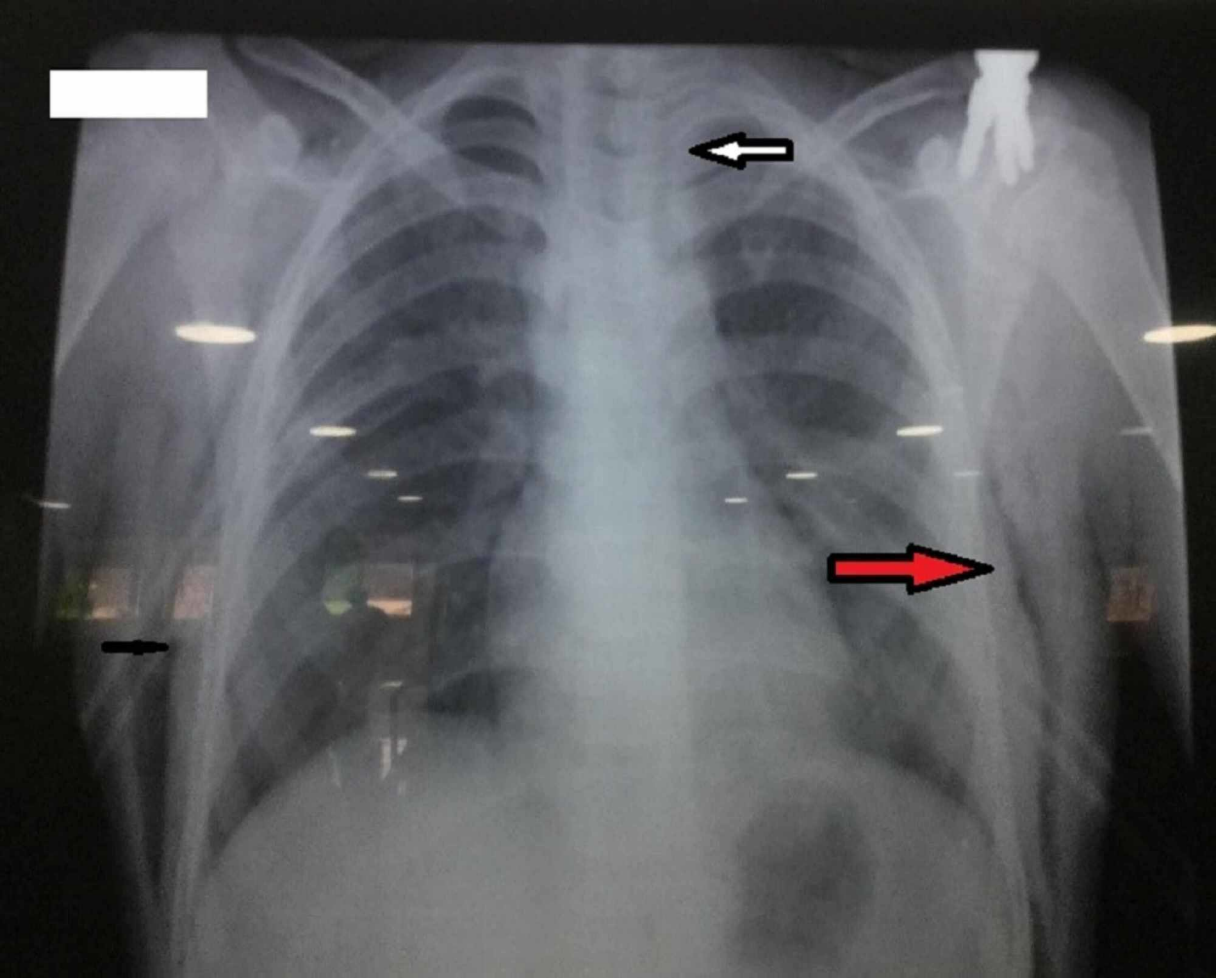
Chest X-ray posteroanterior view showing gas in the mediastinum (white arrow) and subcutaneous emphysema (red arrow)
